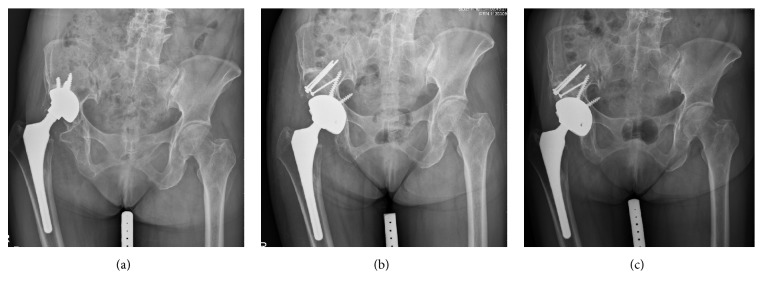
A 61-year-old female patient, with aseptic loosening of right acetabular component. (a) Preoperative radiography showed superolateral cup migration and massive bone defect (Paprosky IIIA). (b) Revision with structural allograft and Trilogy Jumbo cup. (c) Follow-up radiography at 4 years showed stable cup position and good incorporation of allograft.
